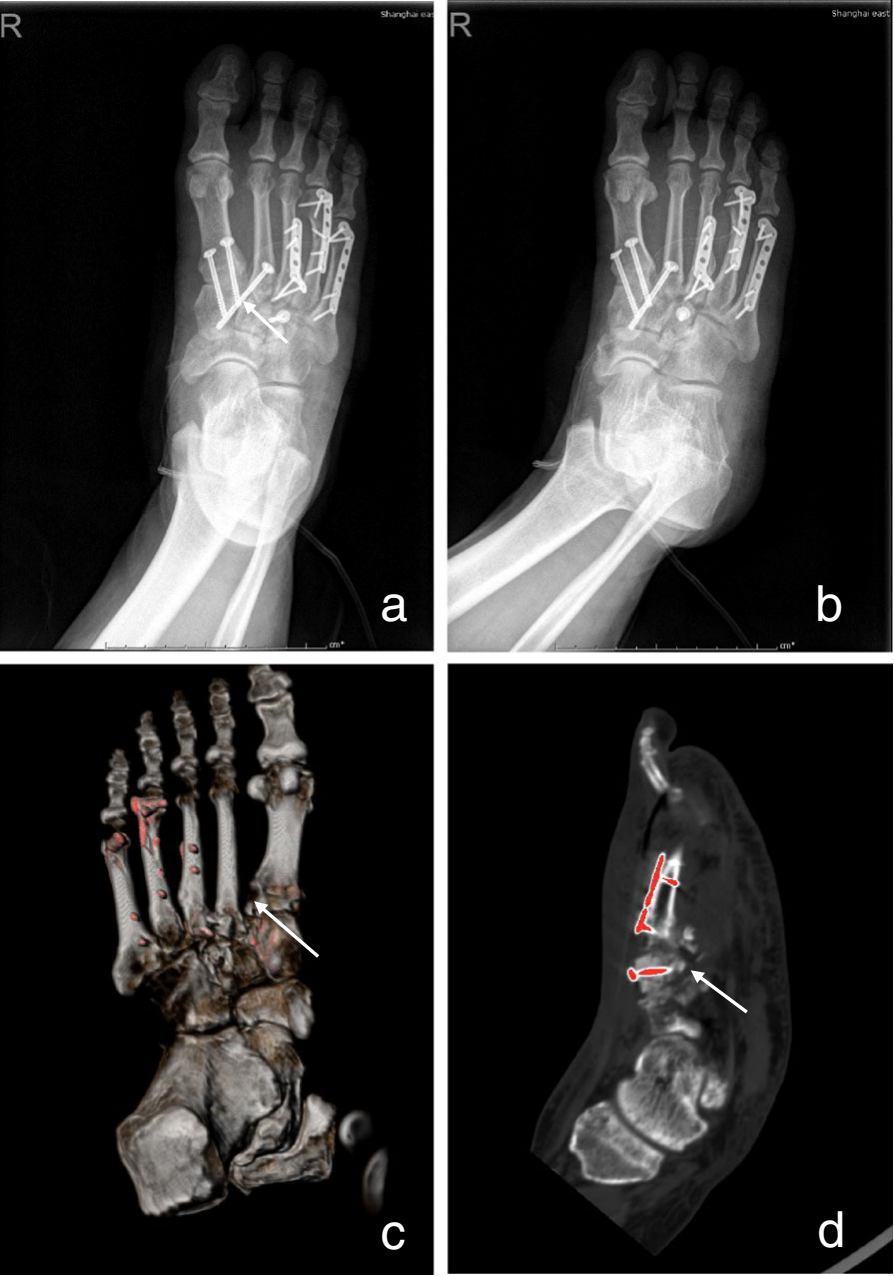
A 53-year-old man with a Lisfranc injury of Myerson classification C1. The postoperative anteroposterior and oblique plain radiograph (a, b) showed the reduction was poor because of the diastasis between the base of the first and second metatarsals was beyond 2 mm, which was also showed clearly in the post-processing CT images in VR mode (d). But furthermore, in the MPR mode of CT images, more fracture fragments at the second and third metatarsal bases were found not reducted with a distance of more than 1 mm (c), while could not be discovered clearly in the plain radiographs
